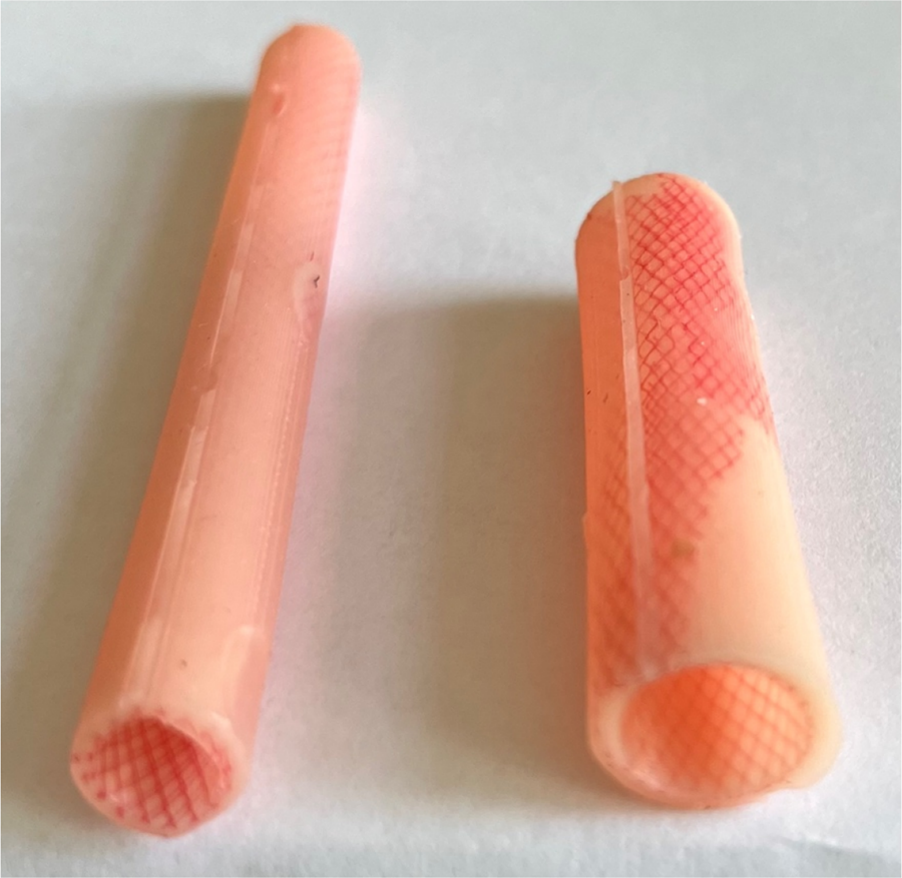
Esophageal stumps are created using silicone, which is poured into a mold generated by a 3D printer. We determined the oesophagus's diameter by measuring the actual width from the initial x-ray image, with a nasogastric tube inserted.
